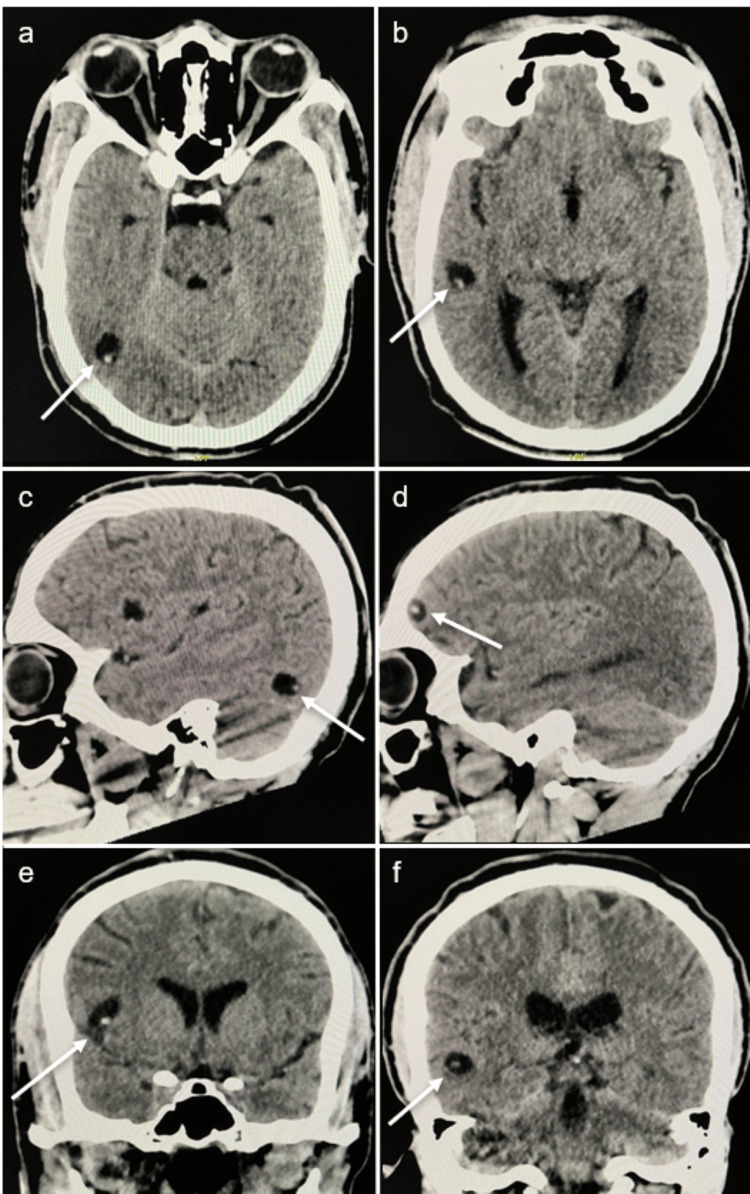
Nonenhanced computed tomography scan of the head showing four intraparenchymal cystic lesions with a small central hyperdense lesion representing scolex with minimal surrounded edema, along with multiple nodular calcifications that are highly suggestive of the vesicular, early colloidal, and calcified stages of intracranial NCC, as marked by the arrows, in the a-b) Axial, c-d) Sagittal, through left hemisphere, and e-f) Coronal views. NCC: neurocysticercosis
